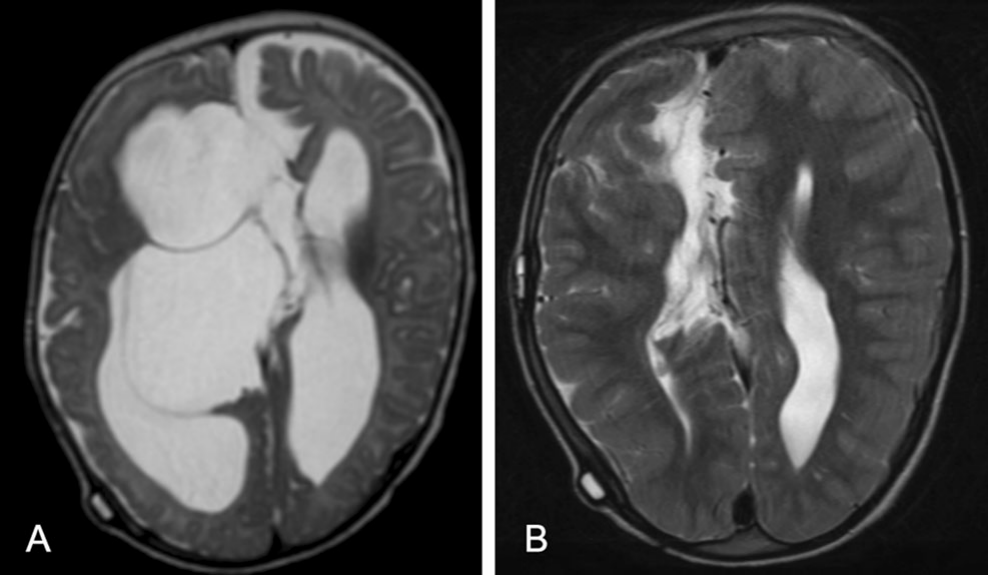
Pre- (a) and postoperative (b) axial T2-weighted MR images of a child with posthemorrhagic cystic ventricular dilatation and no longer functional ventriculoperitoneal shunt
